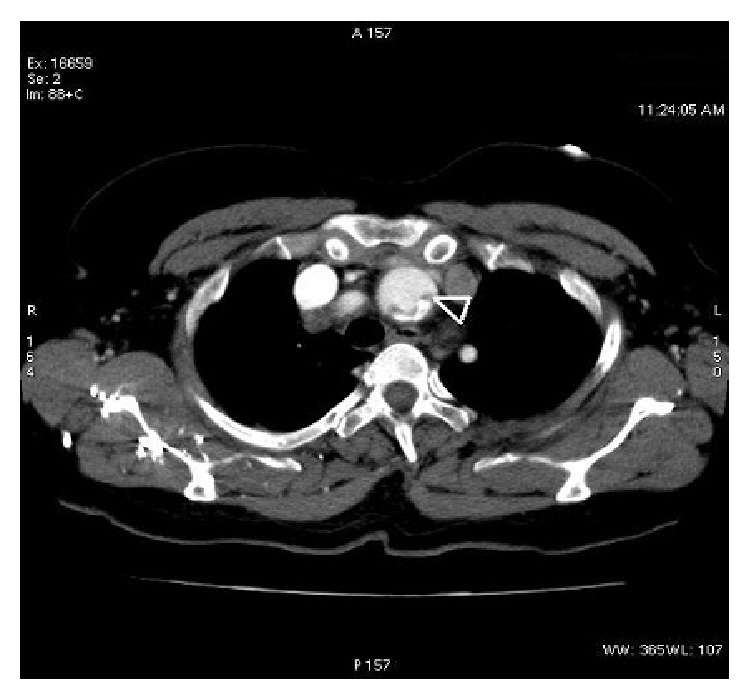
CT scan with contrast enhancement disclosed dissection in the brachiocephalic trunk (arrowhead).
